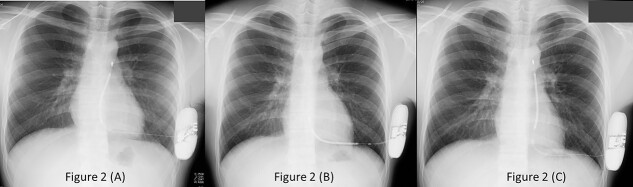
(A) Chest X-ray showing that the extra thoracic lead and generator of S-ICD were first located in the left lateral side; (B) outpatient chest X-ray showed dislodgement of the defibrillation electrode leads in the Para sternum; (C) chest X-ray after replacement of S-ICD using a three-incision technique.
